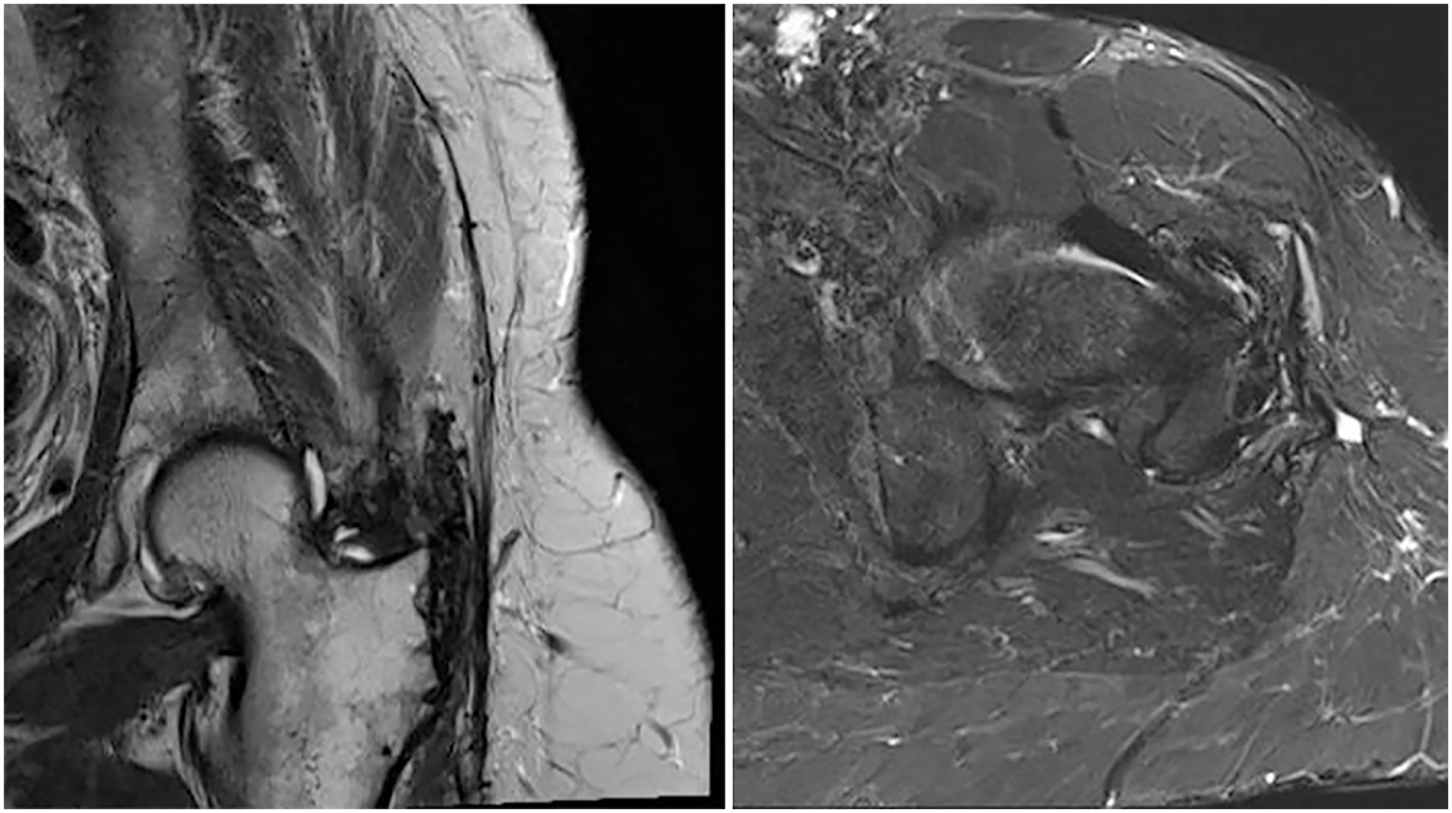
MR image depicting structural integrity of the extraanatomical tendon repair at a follow-up more than 2 years postoperatively.
